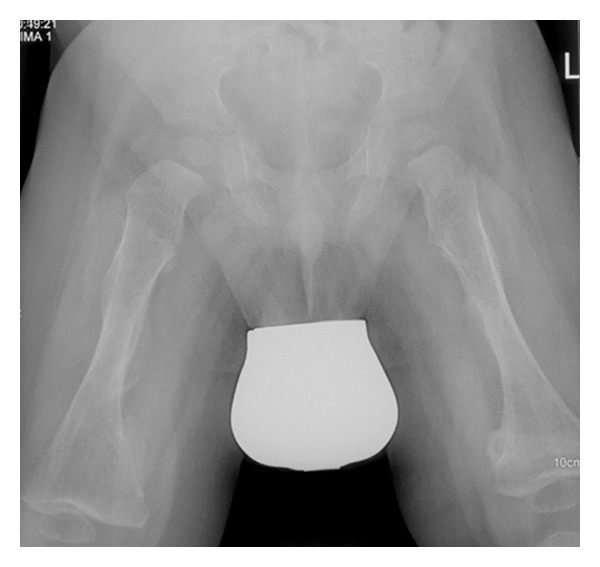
Anteroposterior pelvic and femora radiograph showed dysplastic ischium, short femora, and broad particularly over the distal parts. Calcification of the interosseous membrane was apparent bilaterally associated with overwhelming osteoporosis and the presence of a radiodense band visualized at the growth plate.
